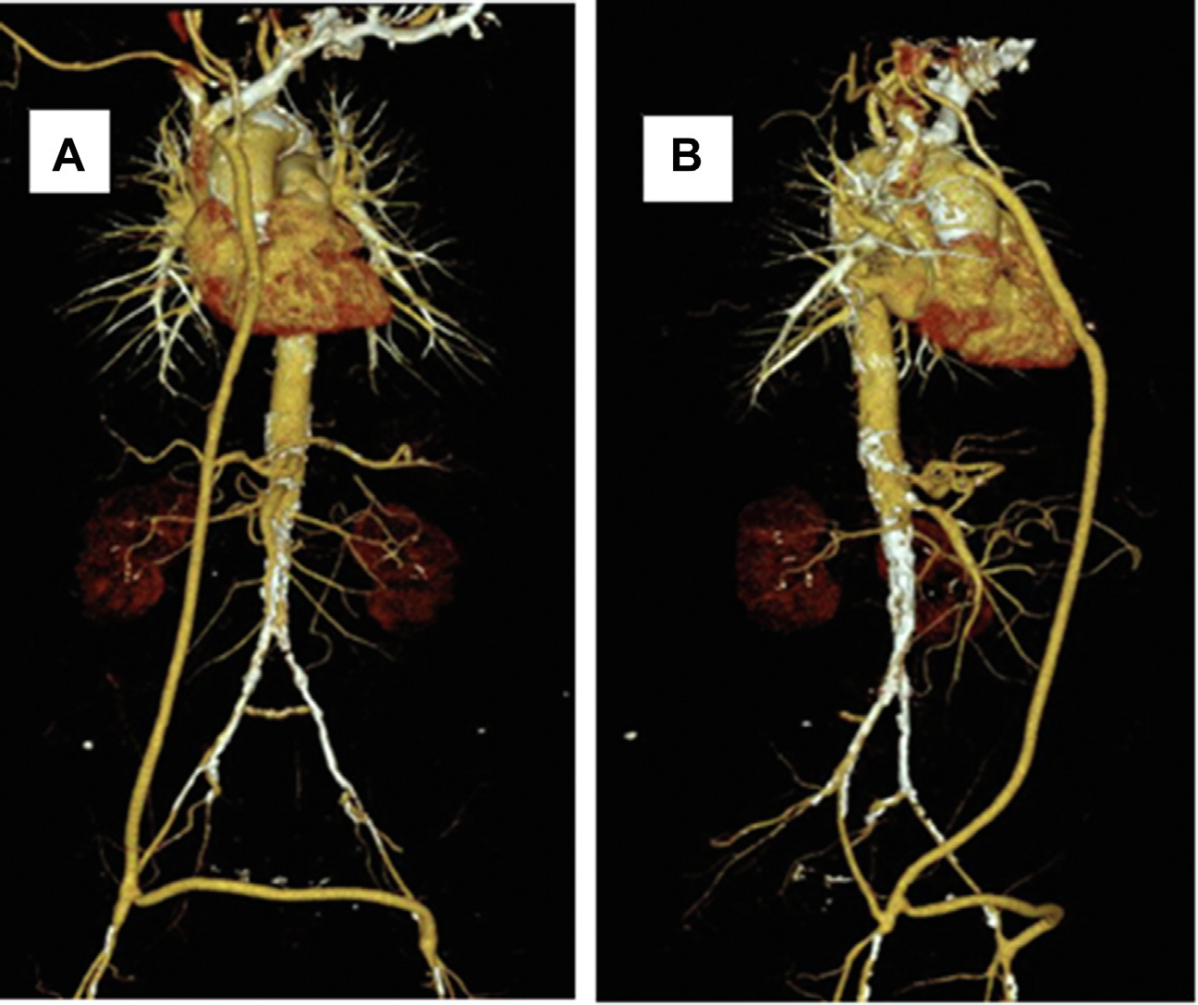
Follow-up computed tomography angiogram at 18 months showing patency of the ascending aortobifemoral (ABF) bypass and carotid artery bypass, without significant stenosis or anastomotic complications.
